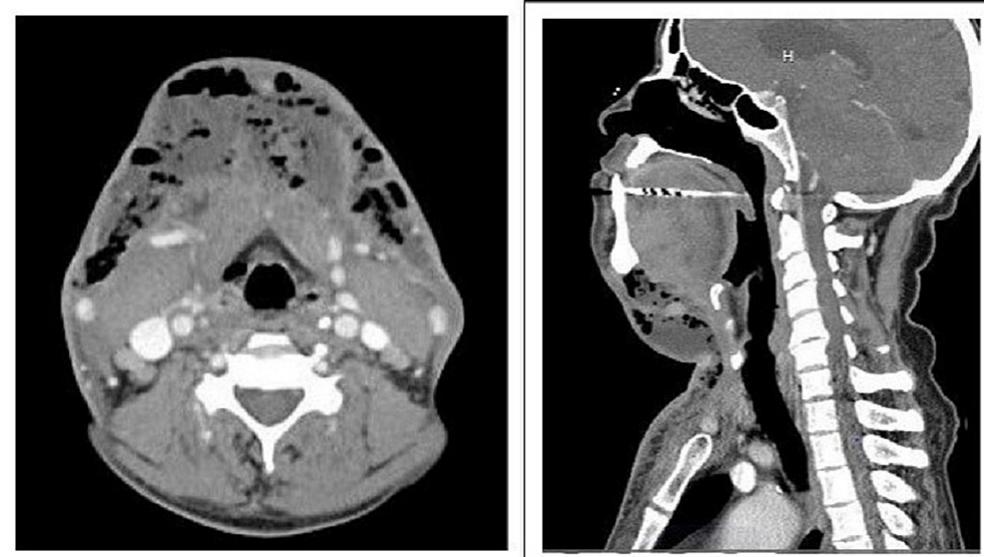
CT scan of neck showing left sublingual space 2.5x0.8 cm fluid collection, submandibular space collection 2x2.1 cm
